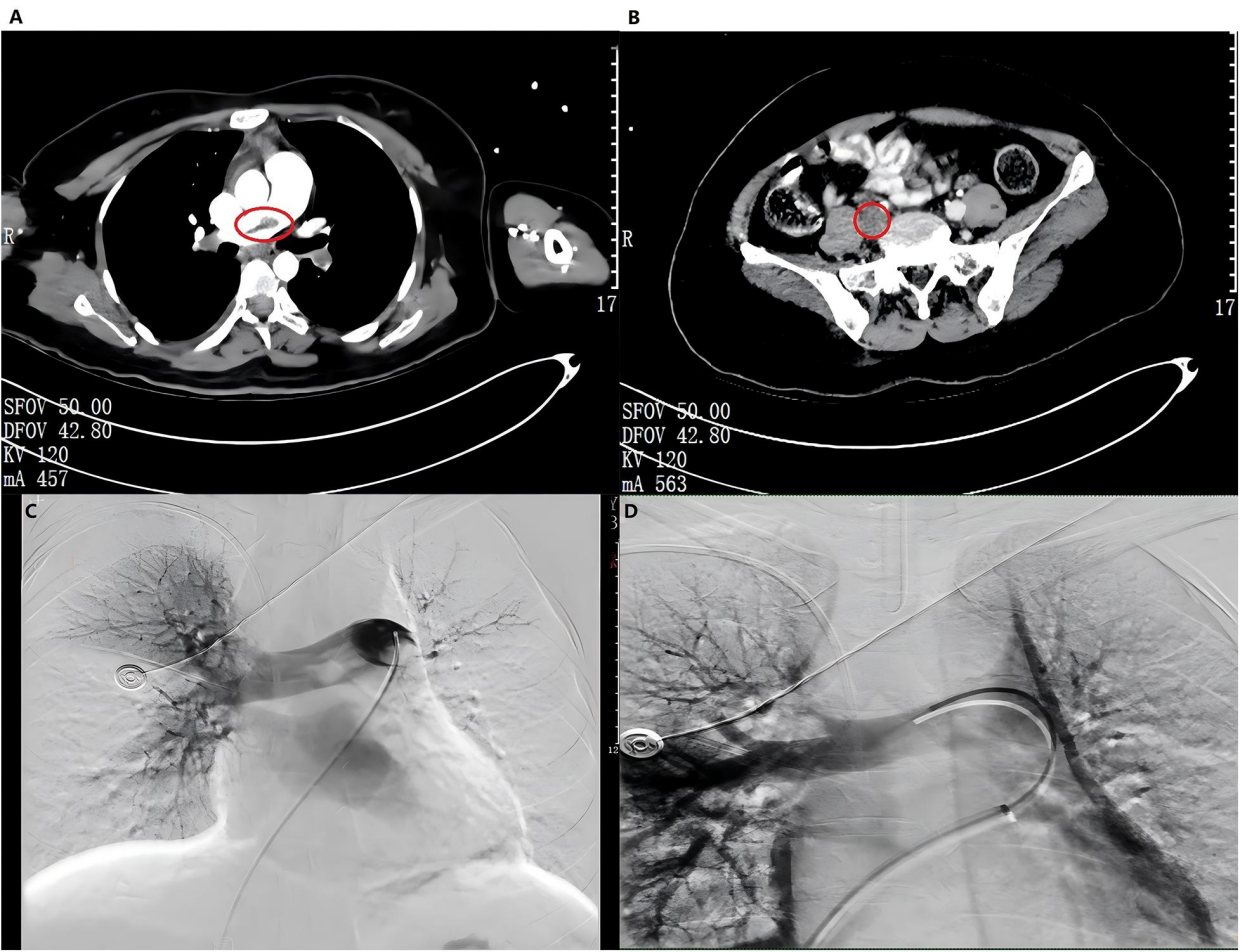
Abdominal/chest computed tomography scans and angiography of the patient. (A) Multiple filling defects in the main pulmonary artery, bilateral pulmonary trunk, and partial pulmonary branches of both lower lobes of the lungs: extensive pulmonary embolism was considered. (B) Multiple filling defects in the right common iliac vein, right internal and external iliac vein, and femoral vein: thrombosis was considered. (C) The angiographic manifestations before interventional thrombectomy. (D) The angiographic manifestations after interventional thrombectomy.
